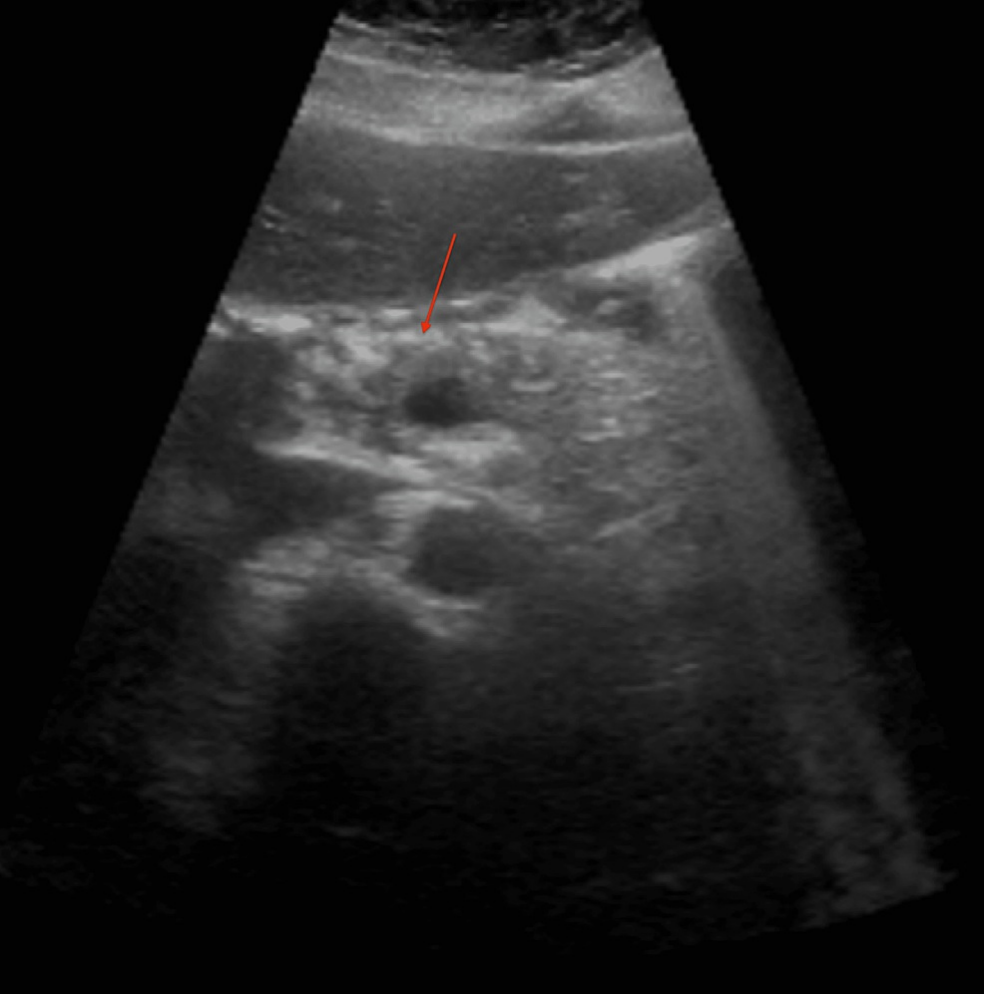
Ultrasound with hypoechoic pancreas consistent with acute pancreatitis.
